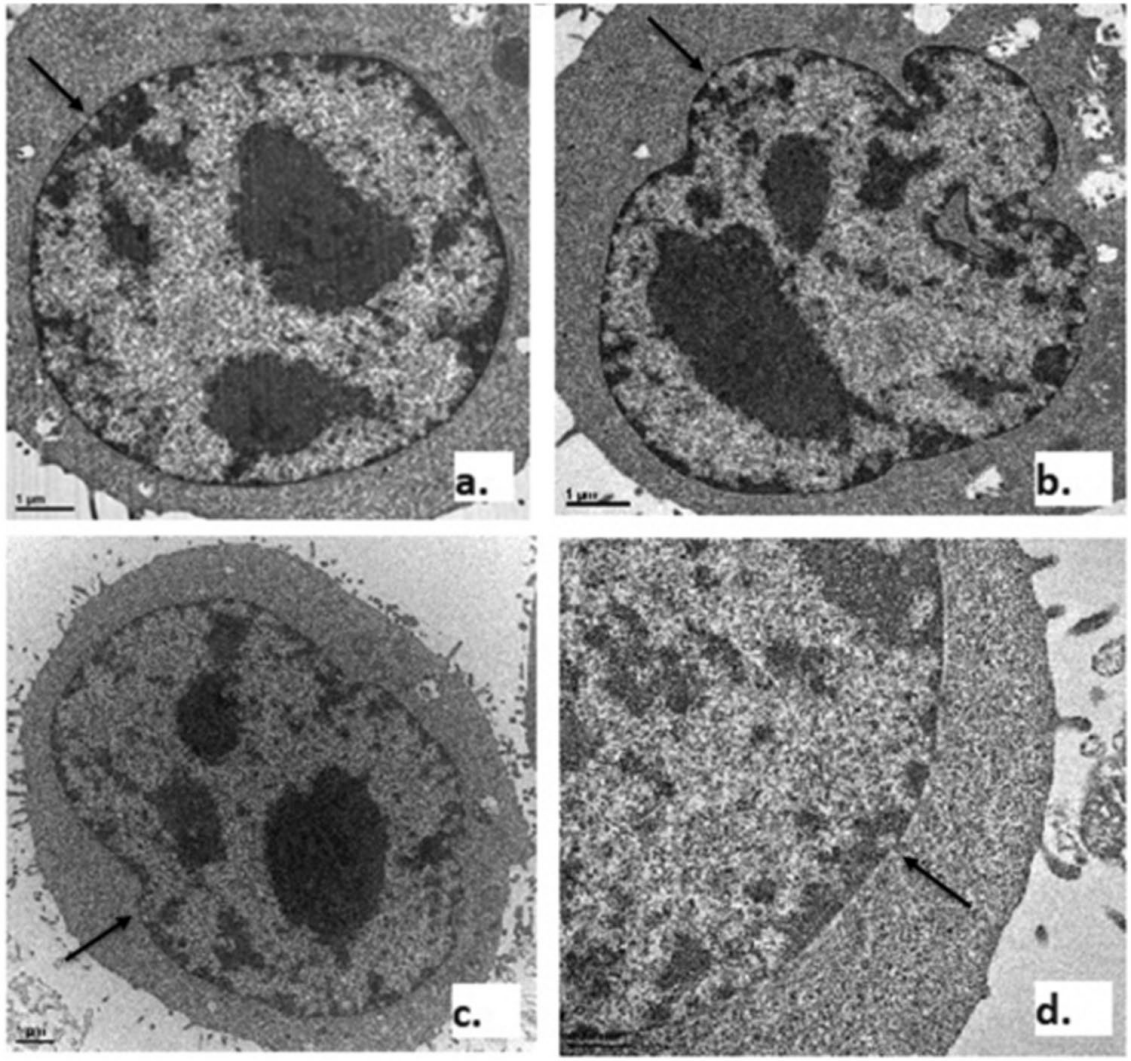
Effect of US on cell membranes (n = 4 per group). Images examples of pores in the cell nucleus in non-treated (x10) (a), doxorubicin (x15) (b), sonicated (x10) (c), and ultrasound + doxorubicin (x30) (d) cells. Pores are pointed by the black arrows.
